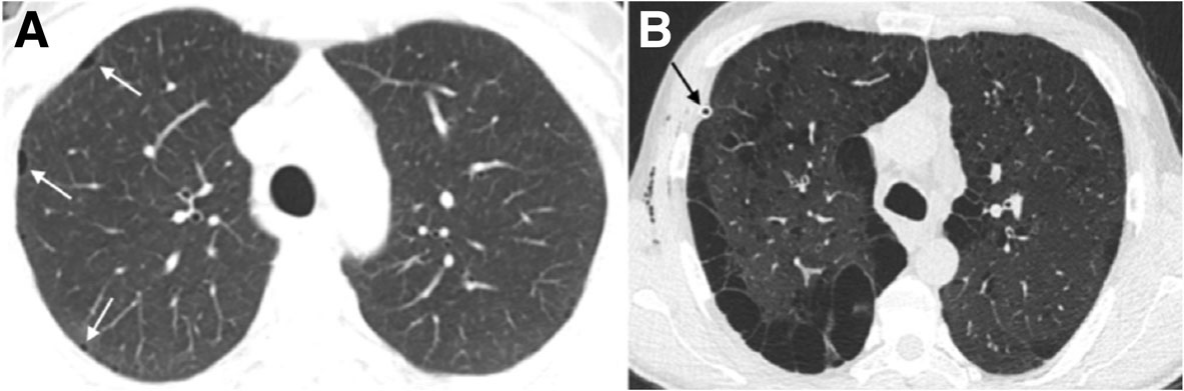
Subtypes of PSE according to the Fleischner Society guidelines on HRCT. a Axial CT shows mild PSE (white arrows) with up to 1 cm juxtapleural well-demarcated lucencies. b Axial CT depicts substantial PSE, defined as cyst-like lucencies or bullae greater than 1 cm adjacent to the pleura. The patient had spontaneous pneumothorax and black arrow depicts a chest drain. Subcutaneous emphysema is also visible in the right thoracic wall
